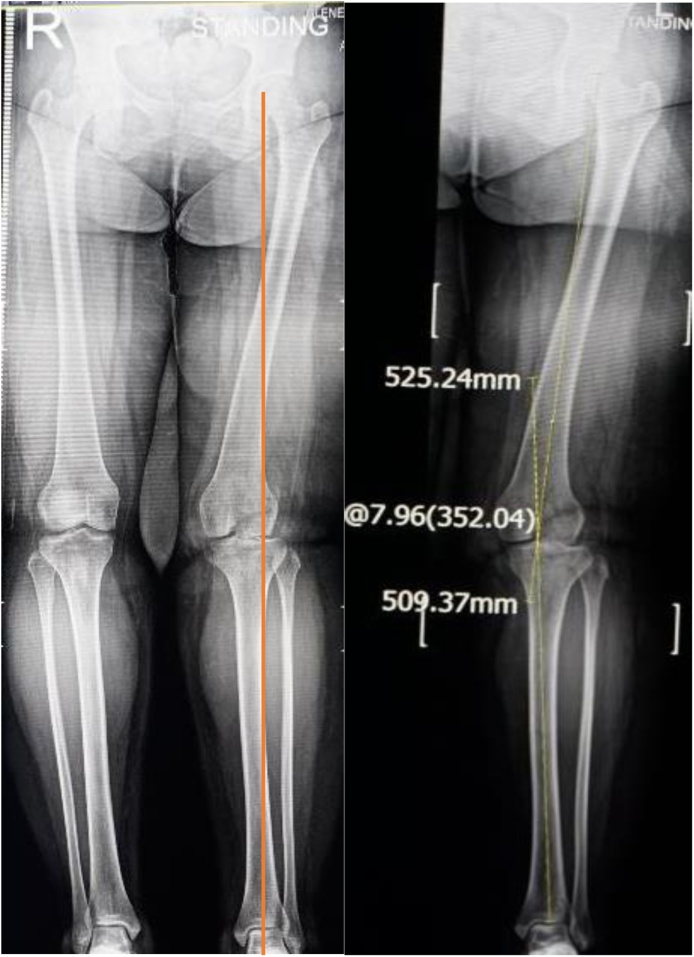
Long standing hip-ankle AP X-ray show the mechanical line angle 110 valgus of left lower extremity.
